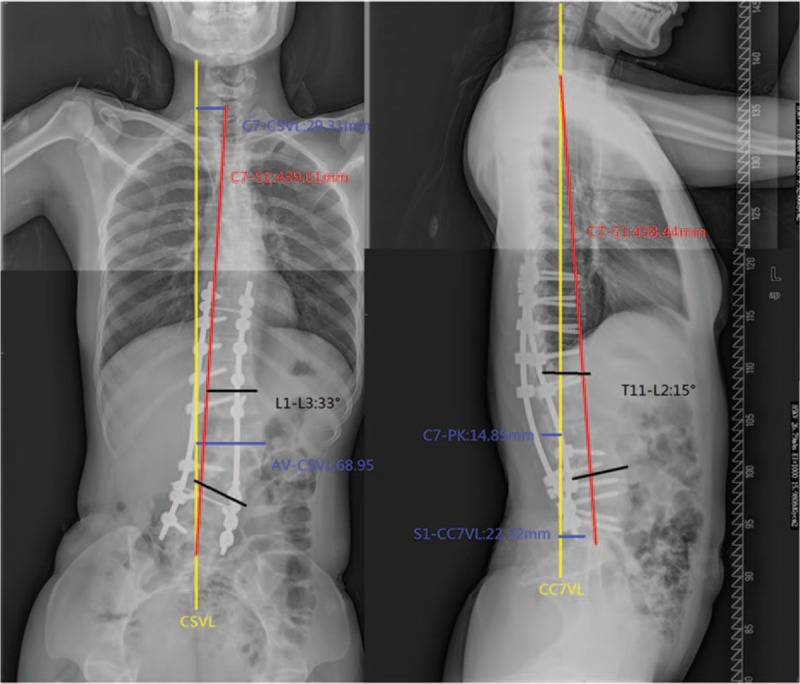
Lateral and anteroposterior X-ray, postoperation.
